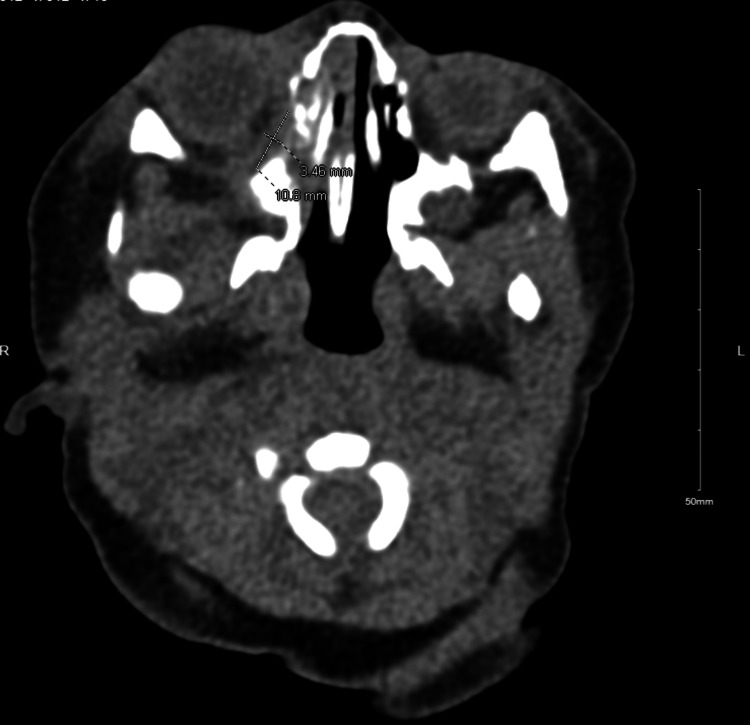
CT Scan Orbits/Sella with contrast shows lentiform collection along the right inferomedial extraconal orbit and associated trace right proptosis
